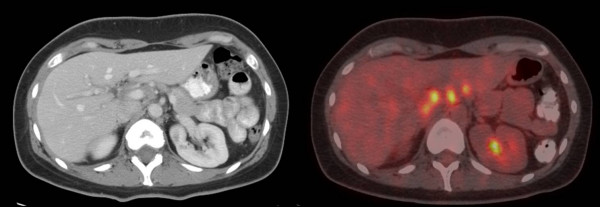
CECT (left) and delayed phase FDG-PET/CT fusion images (right) of a 42 year old female with lung carcinoma showing FDG uptake in periportal/peripancreatic lymph nodes not clearly demonstrated on CECT.
